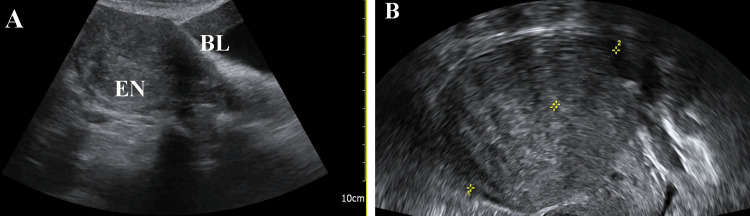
Ultrasound images of the uterus A shows the uterine ultrasonography; the uterus appears uniformly enlarged and symmetrical (68.8×64.3×55.7 mm) with a thickened muscle layer and heterogeneous echo, and the endometrium is centrally located with unclear demarcation from the muscle layer. B - The muscular layer of the anterior wall was thicker than that of the posterior wall, about 42.1 mm (yellow crosshair 1) and 29.3 mm (yellow crosshair 2) EN, endometrium; BL, bladder
